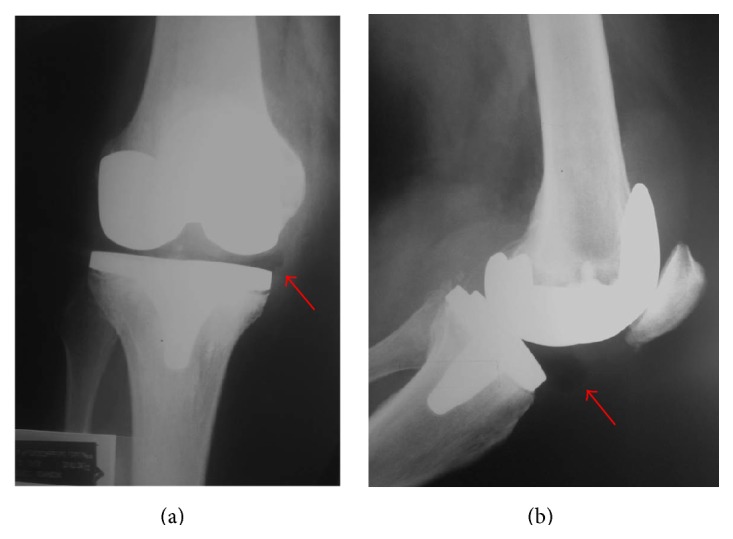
Anteroposterior (a) and lateral (b) radiographs of the right knee demonstrating a sign of anterior tibial insert dislocation.
